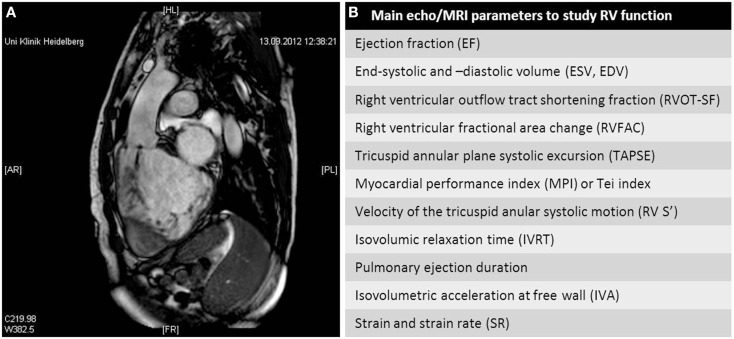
(A) Magnetic resonance imaging scan of a patient with ccTGA and dilatation and dysfunction of the systemic right ventricle. (B) Main echocardiographic and MRI parameters to study the function of the right ventricle.
